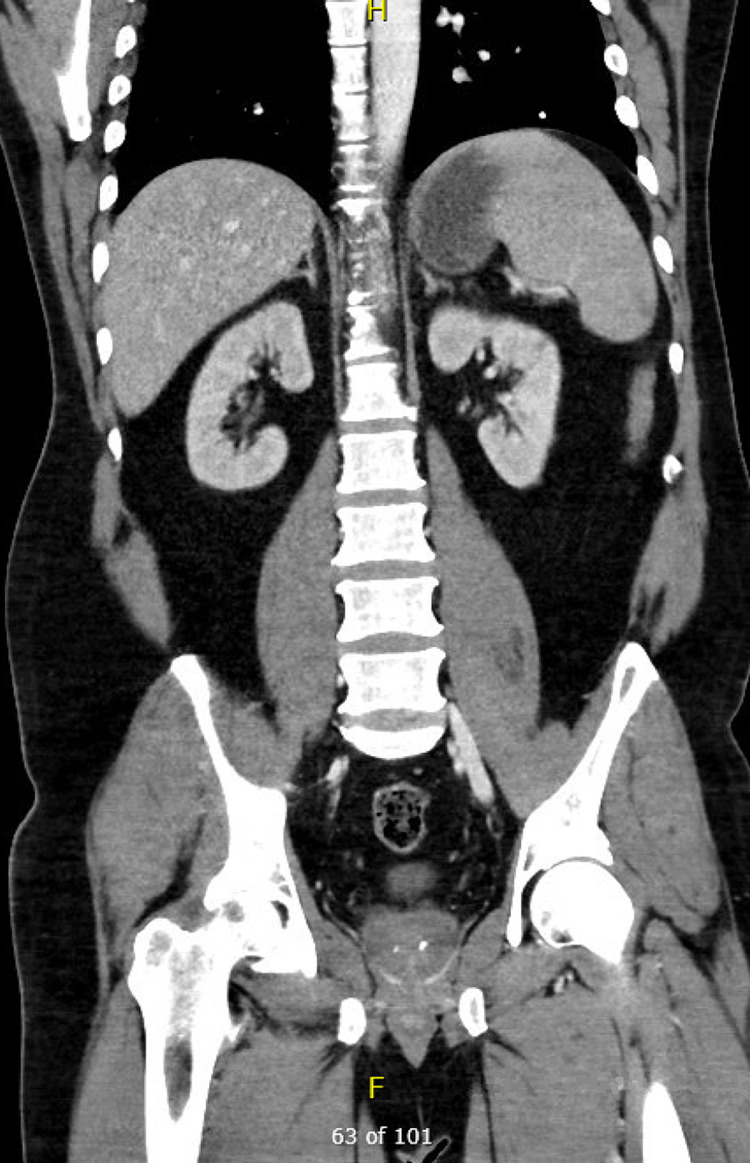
CT abdomen and pelvis showing mild splenomegaly
